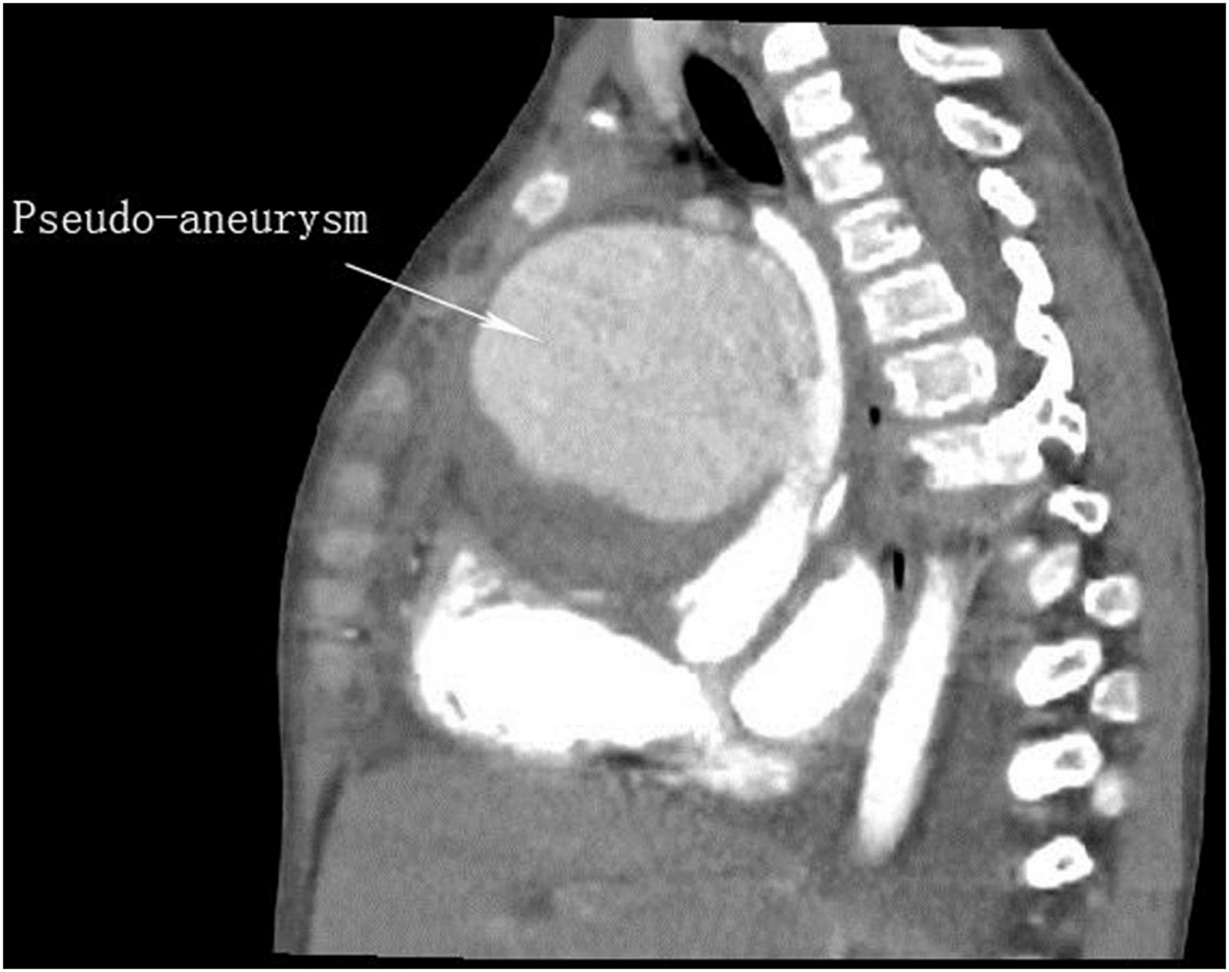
Sagittal view of contrast enhancement CT image shows a large pseudo-aneurysm is bound up with the ascending aorta.
